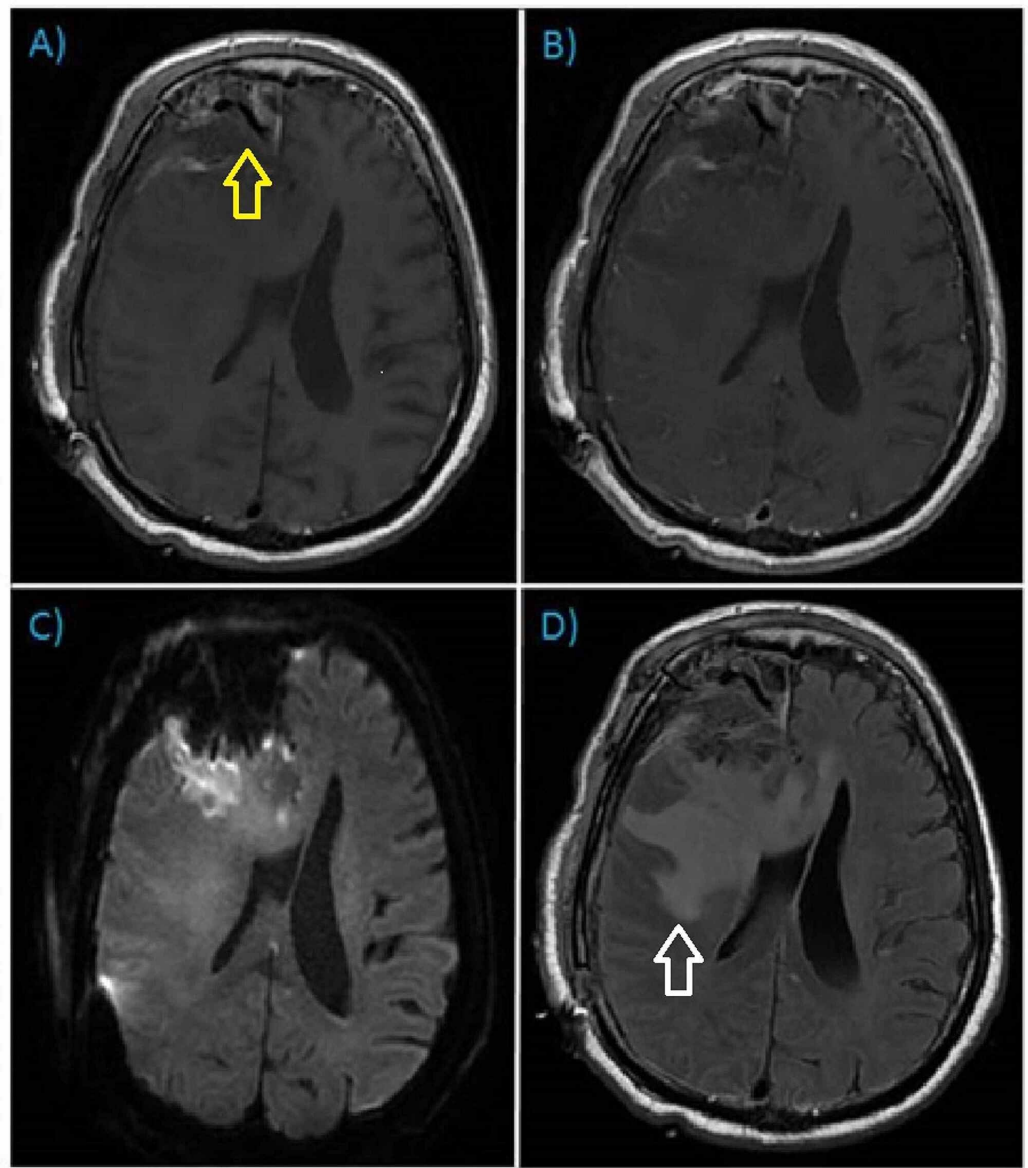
Post-operative MRI brain with and without gadolinium Post-operative MRI brain with and without contrast, axial orientation. A) T1-weighted pre-contrast; B) T1-weighted post-contrast; C) Diffusion Weighted Imaging (DWI); D) T2-weighted Fluid Attenuation Inversion Recovery (FLAIR). Post-surgical changes evident (yellow arrow) with re-demonstration of vasogenic edema (white arrow) and no residual enhancing mass.
